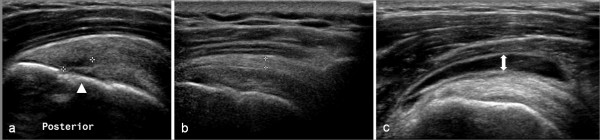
Ultrasound scan images of subacromial bursa and supraspinatus pathology. a) hypoechoic region (between calipers) indicating an intrasubstance tear within posterior fibres of supraspinatus (longitudinal view) overlying the head of humerus (white arrowhead); b) thickened subacromial bursa (calipers); c) bunching of the SAB (white arrow) under the acromion during dynamic abduction.
